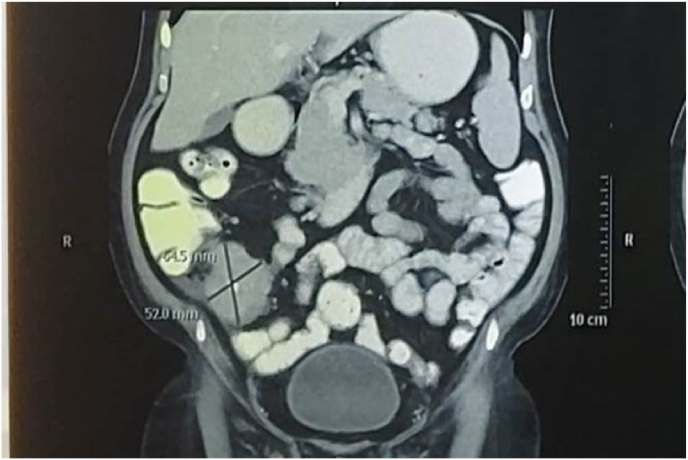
CT scan showing mass lesion in caecum (Sagittal section).
